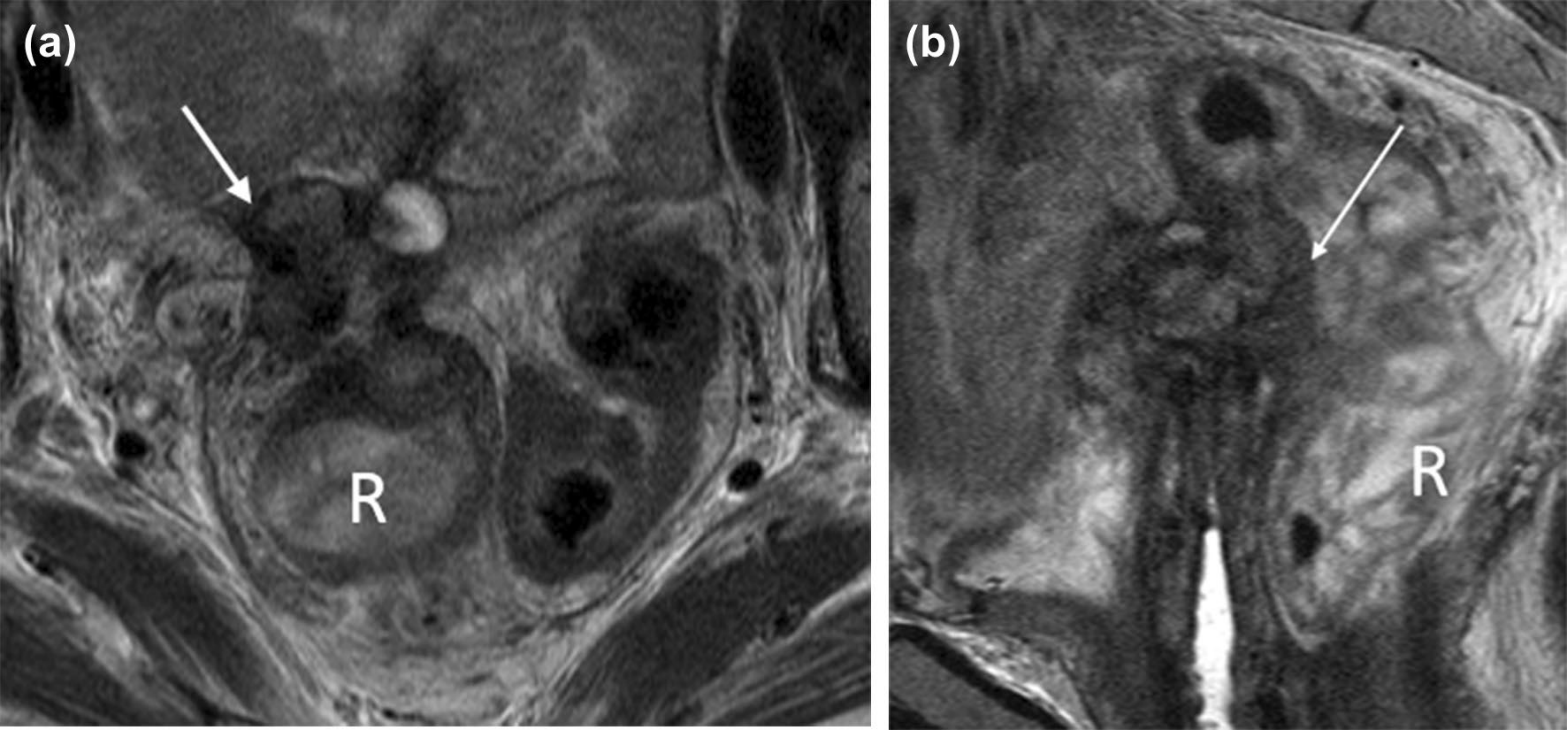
62-year-old woman with FIGO stage 4 vaginal cancer. a Axial T2-weighted MR image shows a complex soft tissue mass (arrow) with a cystic component at the superior edge of the vaginal cuff. The mass abuts several small bowel loops, with apparent tethering of surrounding structures. b Sagittal T2-weighted MR image shows that the mass invades the upper rectum (arrow). There is circumferential wall thickening of the upper rectum and sigmoid colon. R = rectum
